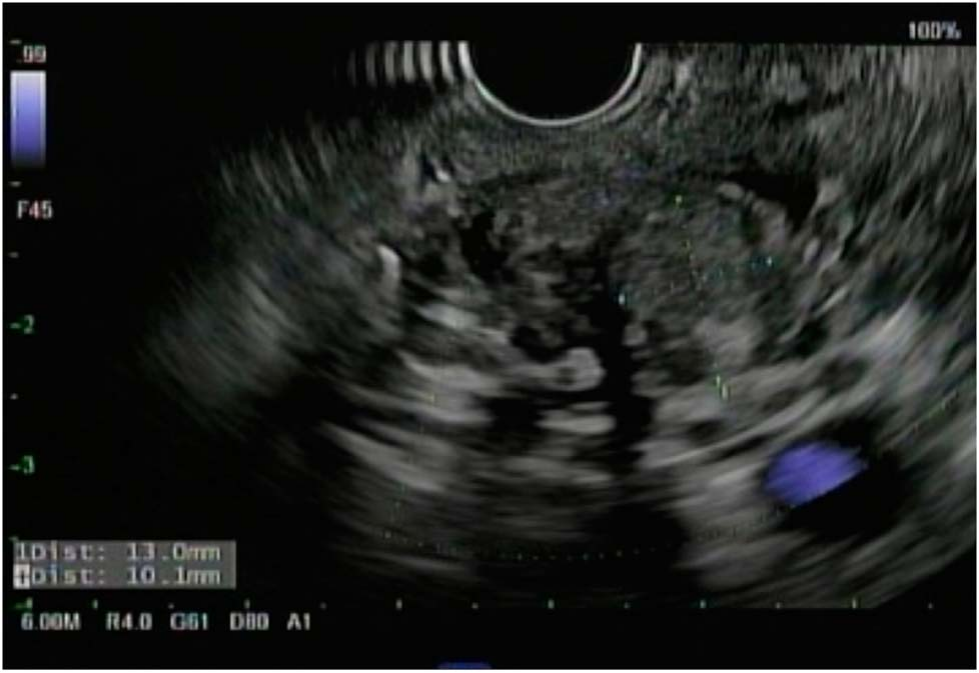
Endoscopic ultrasound showing a 15 mm mass in the pancreatic head.
